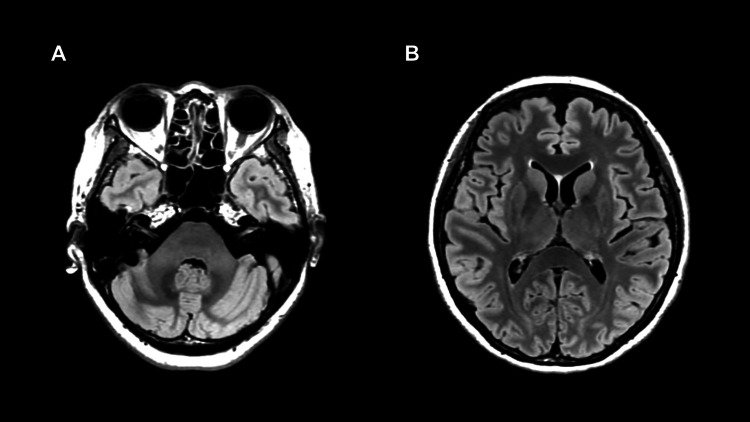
Brain MRI Axial brain fluid-attenuated inversion recovery MRI images. (A and B) The images show no apparent abnormalities. All imaging studies were performed before initiation of steroid therapy.
